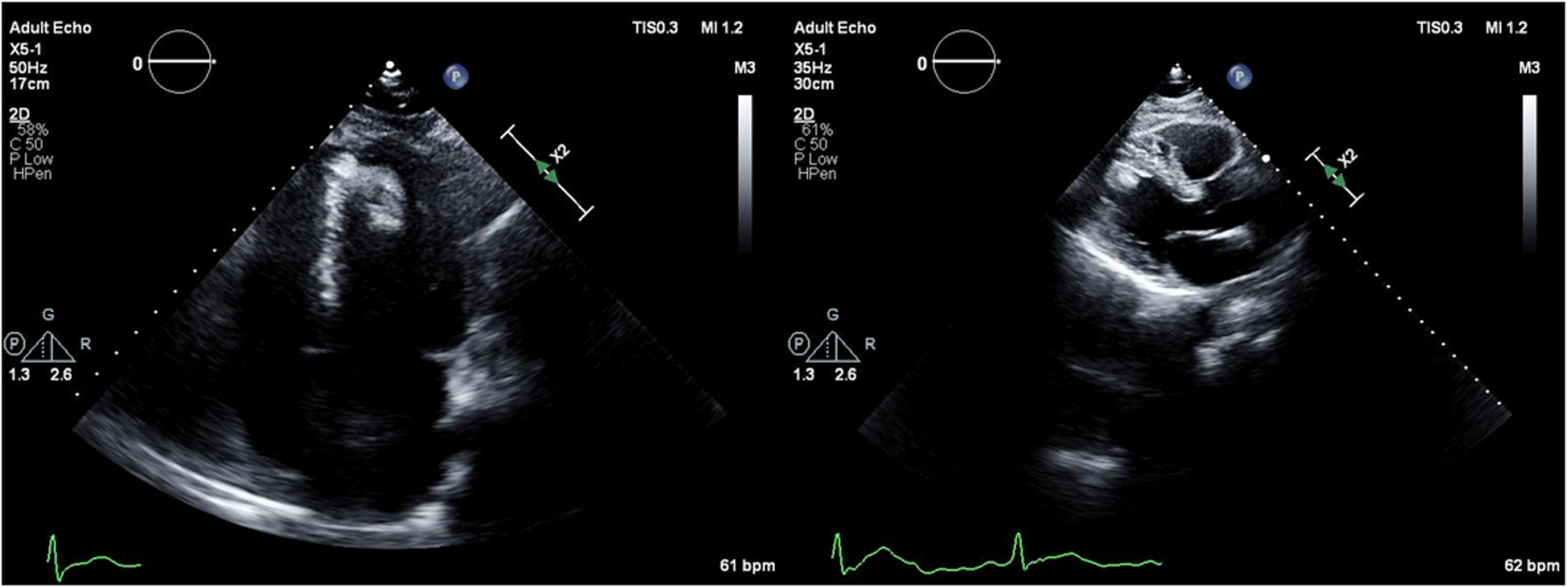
Transthoracic echocardiogram (long and short axis view) showing a pendunculated lobular mass
